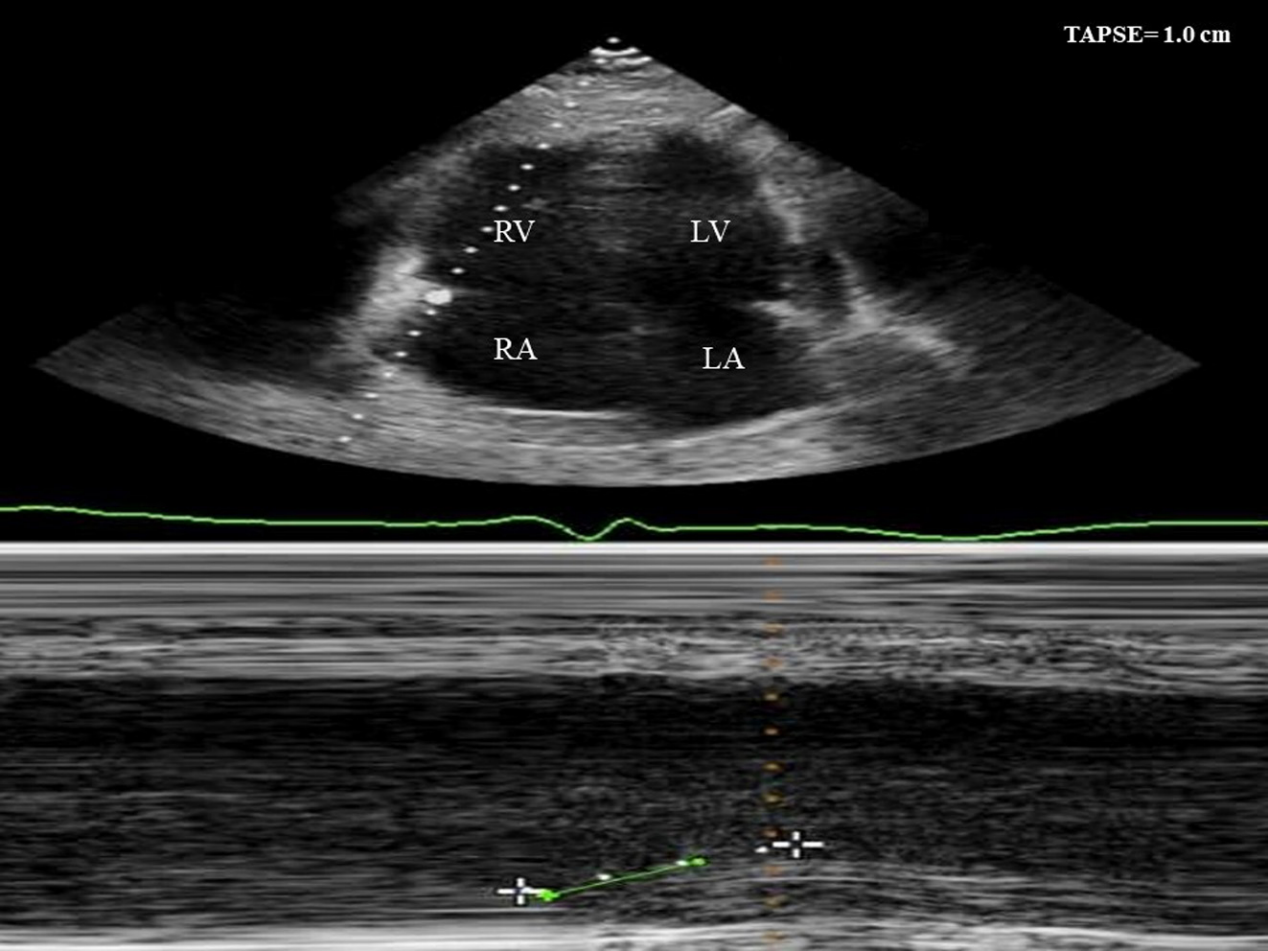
Echocardiography showing biventricular systolic dysfunction with markedly dilated right ventricle (RV) with reduced RV systolic function (tricuspid annular plane systolic excursion (TAPSE) = 1.0 cm).
